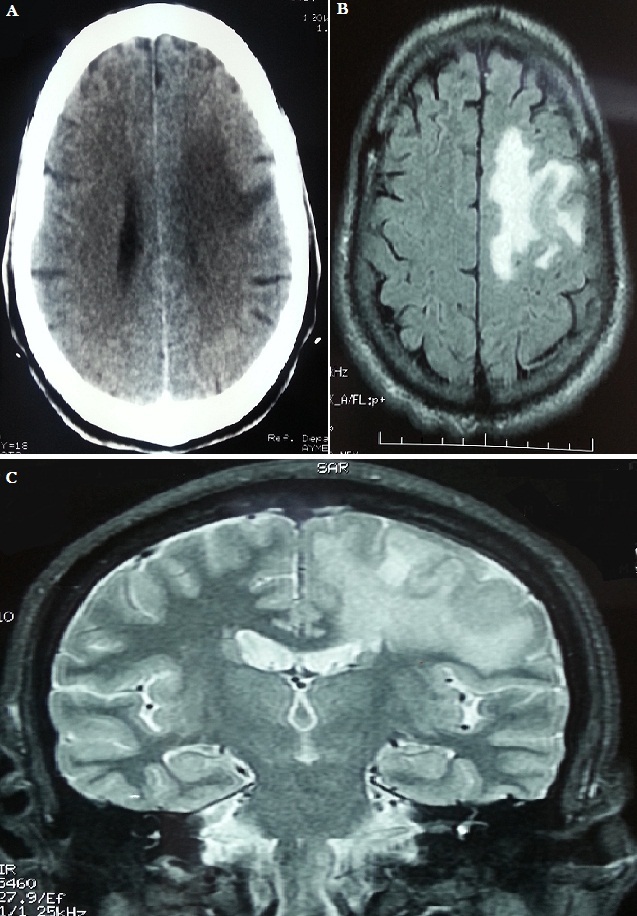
A) brain CT scan: a left frontal tumor process with peri-lesional oedema; B) axial brain MRI: a left posterior frontal peripheral meningioma with intralesional bleeding and significant perilesional oedema with mass effect on the ipsilateral lateral ventricle; C) frontal brain MRI section: a left posterior frontal peripheral meningioma with intralesional bleeding and significant peri-lesional oedema with mass effect on the ipsilateral lateral ventricle
